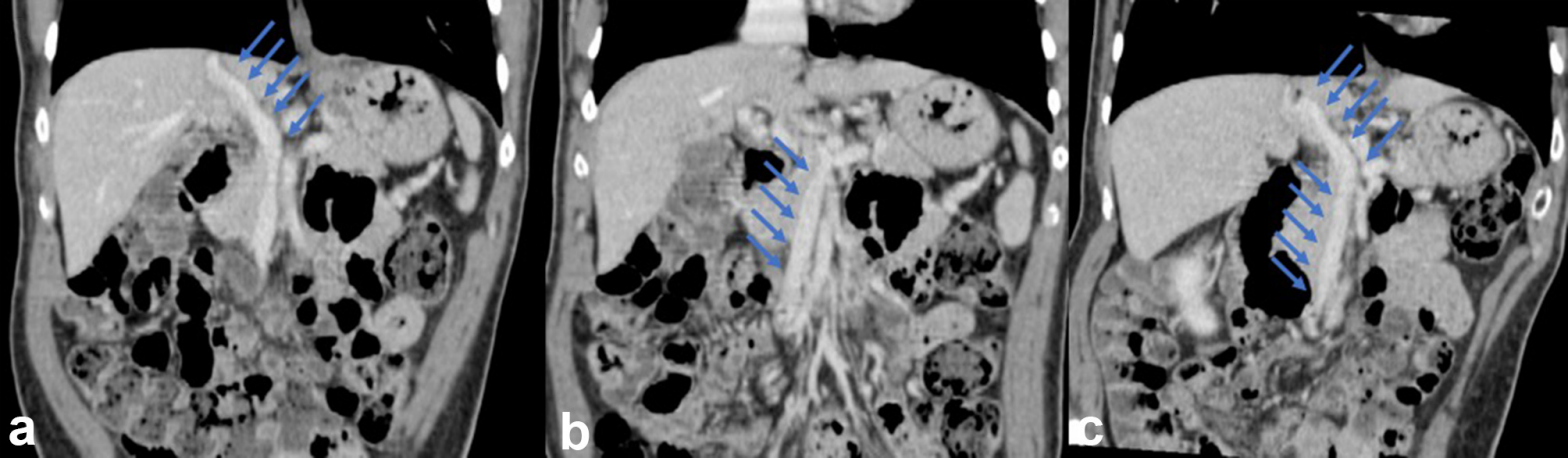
Contrast-enhanced abdominal CT scan. Coronal images showed the presence of an abnormal short portal vein, with a systemic drainage in the inferior cava vein (a), and the superior mesenteric vein and splenic vein draining in the abnormal portal vein (b and c), as depicted by the arrows.
